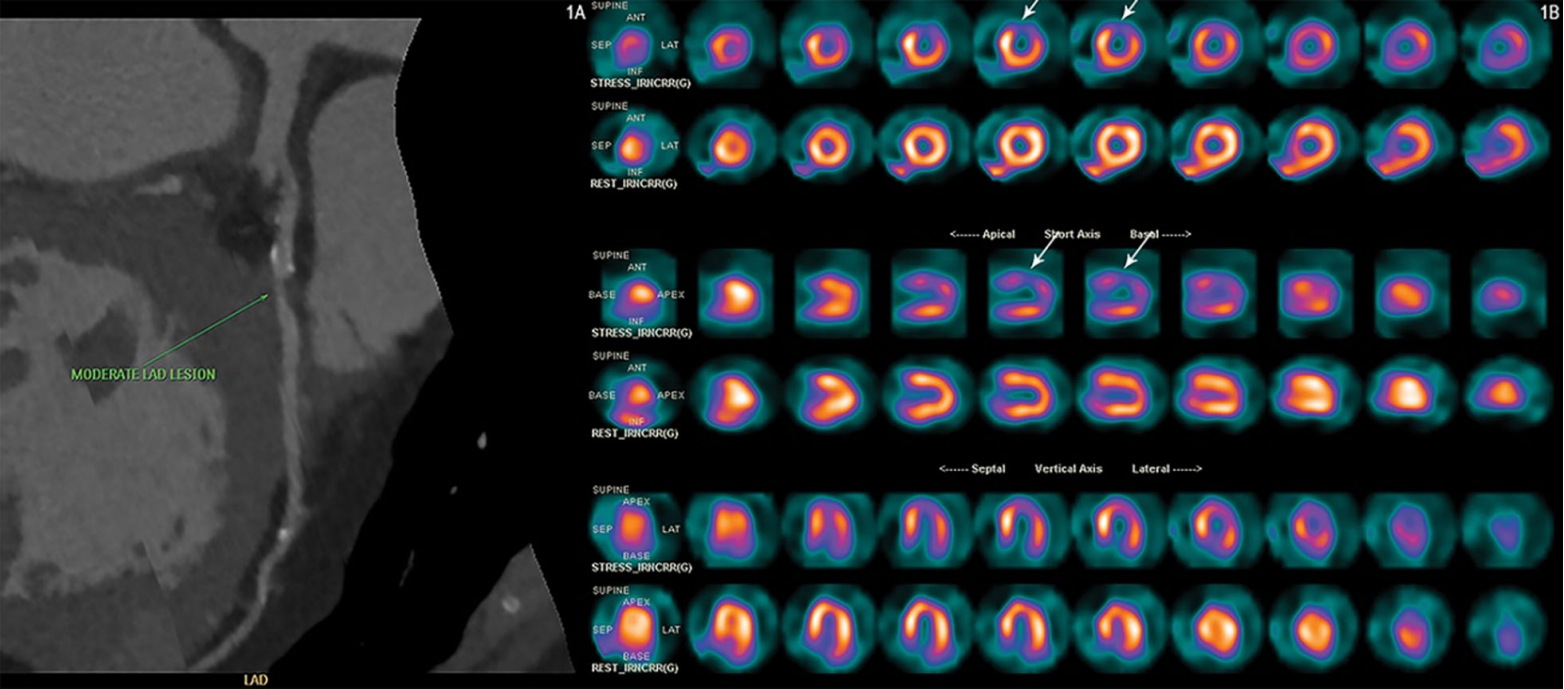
A 68-year-old diabetic male patient with atypical symptoms underwent CCTA which showed a calcium score of 640 Agatston and a partially calcified proximal LAD plaque, causing moderate stenosis (1A -LAD curved multiplanar reconstruction). Subsequent exercise stress MPI revealed severe ischemia (*arrows*) in the anterior wall, antero lateral region and apex (1B). *MPI* myocardial perfusion imaging, *CCTA* coronary CT angiography, *LAD* left anterior descending coronary artery
